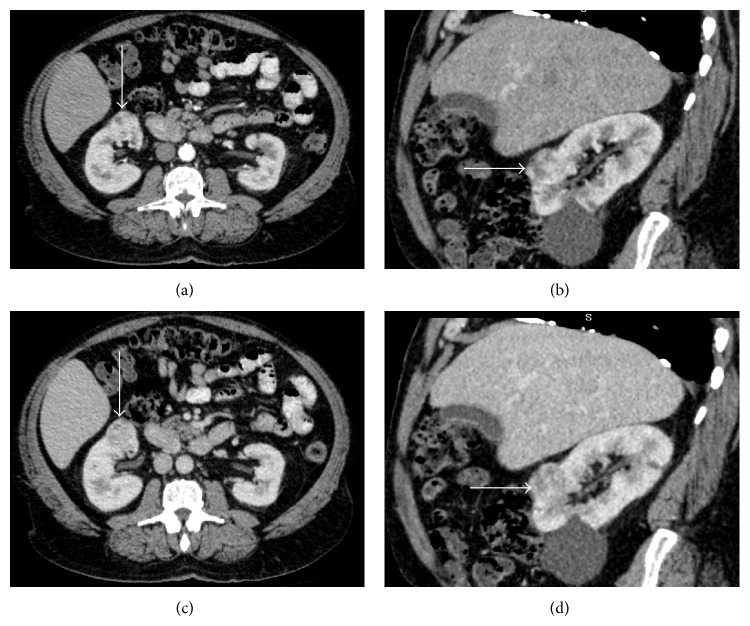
Clear cell carcinoma. ((a)-(b)) Contrast-enhanced CT scans in arterial phase. ((c)-(d)) Portal phase CT scans. The tumor with inhomogeneous aspect is recognizable at the anterior portion of the right kidney (arrows).
